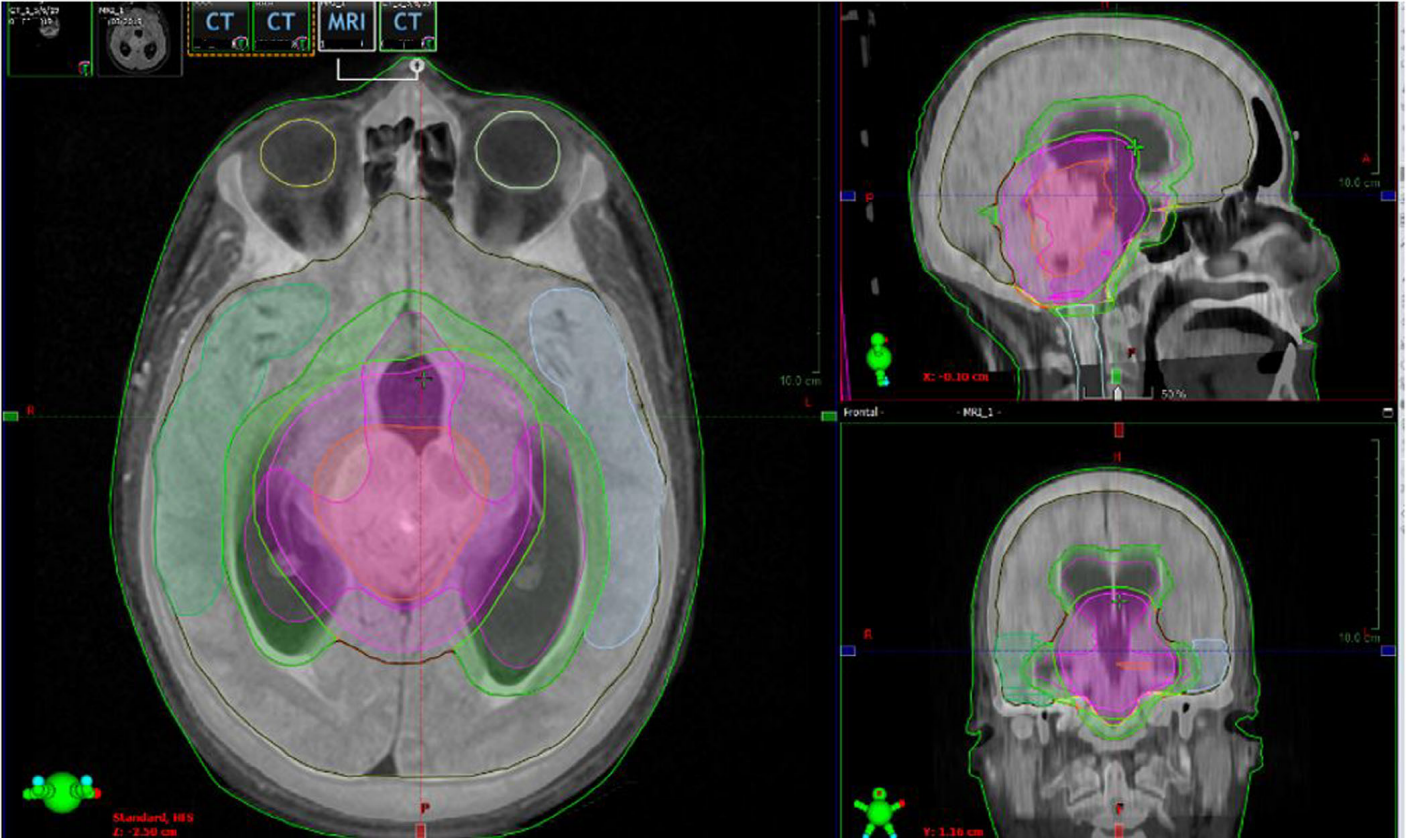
Radiotherapy planning process: computed tomography without contrast merged with the initial MRI
